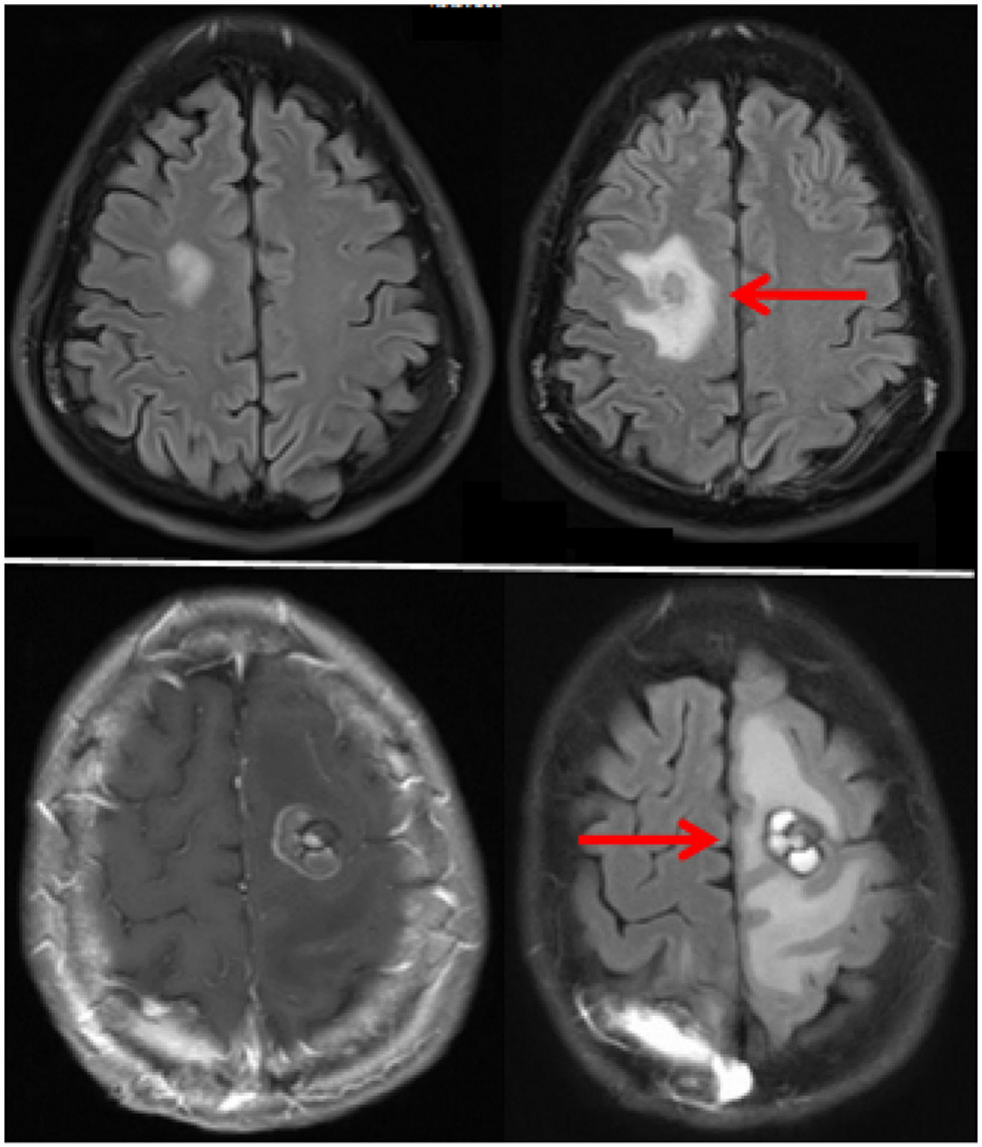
MRI FLAIR images of two patients with perilesional while receiving pembrolizumab. The top and bottom frames represent the two separate patients. Images prior to therapy are on the left and after therapy on the right.
